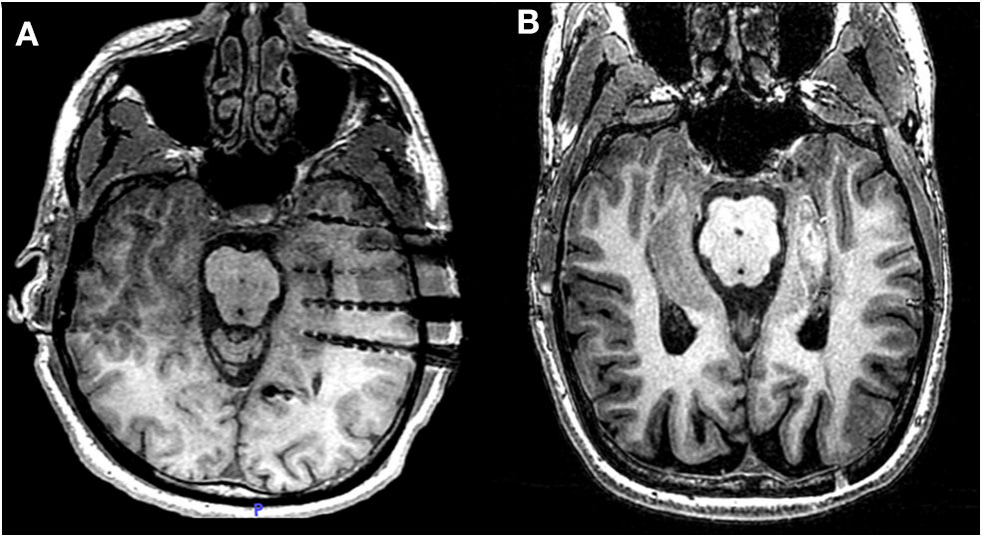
Laser ablation after sEEG investigation. (A) Post-operative MRI of patient 2 showing three electrodes covering the head, the body and the tail of the hippocampus. From each electrode lead, two contacts are recording from hippocampal parenchyma. (B) Post-ablation MRI of the same patient, showing the extent of the lesion across the MT longitudinal axis.
